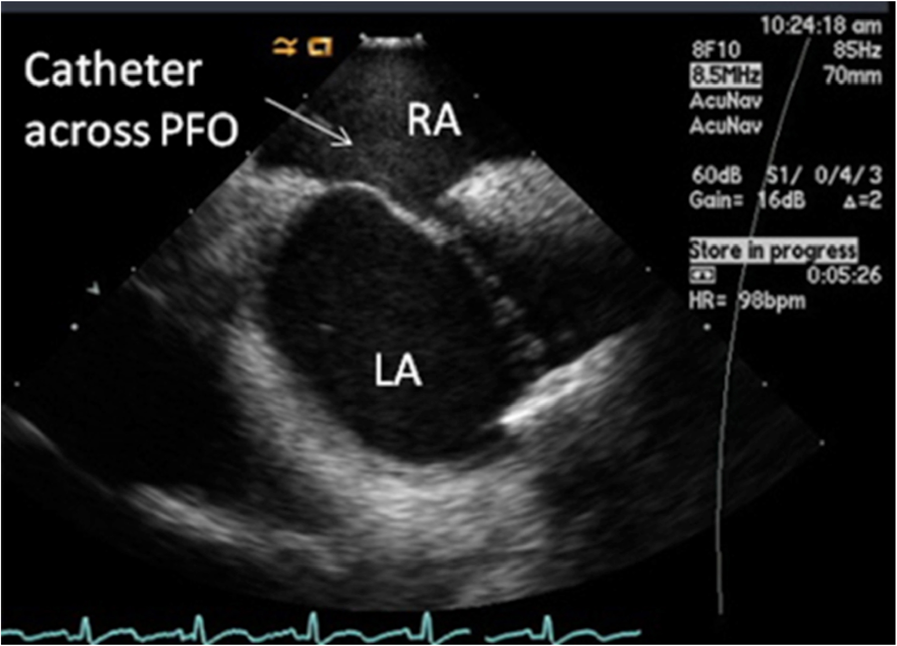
Intra-cardiac echocardiography. The guidewire and catheter can be seen in the heart, passing from the right atrium (*RA*), through the patent foramen ovale, into the left atrium (*LA*). The *bright white area* (echo dense) on the wall of the *LA* opposite the *RA* is the occlude device with the guidewire attached [29].
